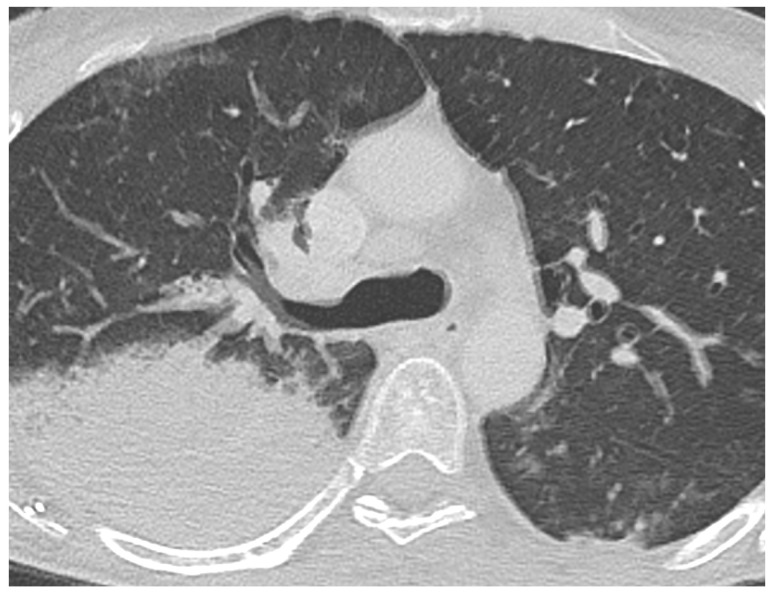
Chest CT in an elderly patient with *Streptococcus pneumoniae* infection: unilateral homogeneous consolidation in the right lower lobe, consistent with lobar pneumonia. Representative image from the anonymized institutional radiology archive of University Hospital Frankfurt.
